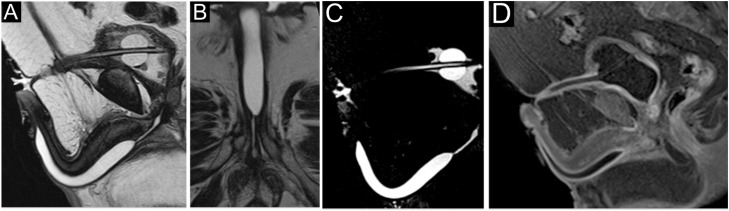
Seventy-year-old male patient with posterior urethral stenosis showing a ring-like appearance secondary to postoperative changes after radical prostatectomy. T2-weighted images (A–D) demonstrate a contrast-enhanced hypointense area consistent with spongiofibrosis at the stenotic segment.
